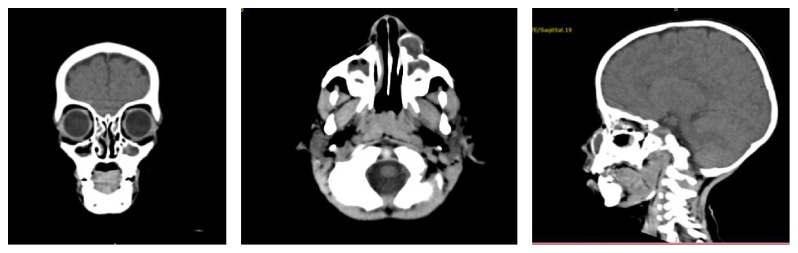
Coronal axial and sagittal CT: odontogenic myxoma left maxilla.
